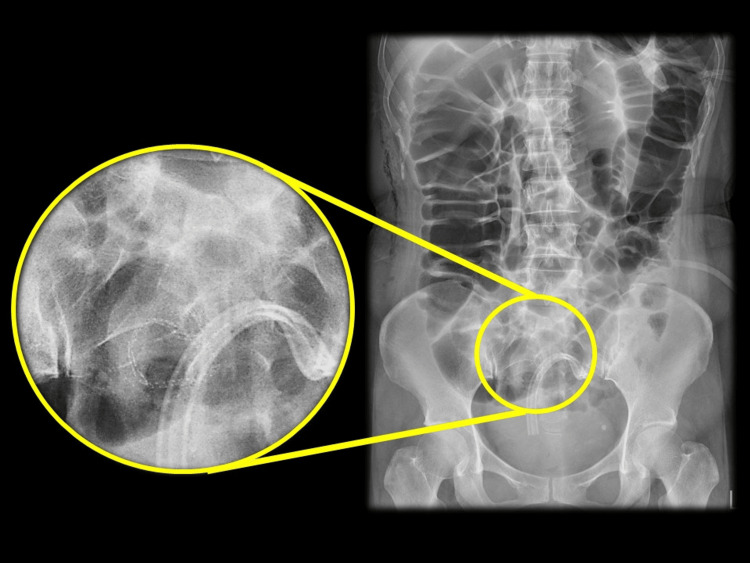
The abdominal X-ray. The abdominal X-ray revealed a significant amount of bowel gas originating from the small bowel to the descending colon and ended in anastomosis.
